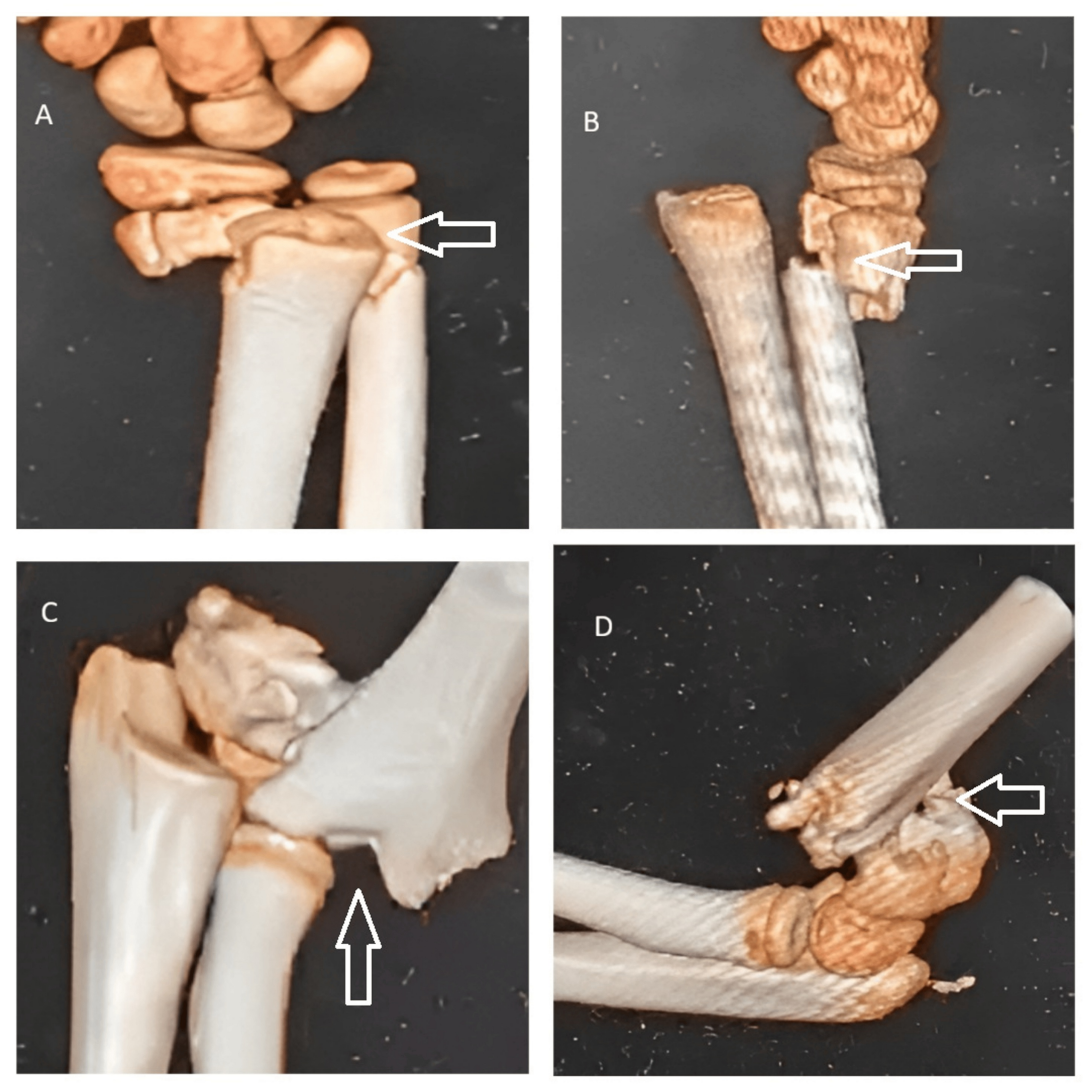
Preoperative CT images of the left elbow and wrist demonstrating fracture configuration Preoperative CT scans of the left upper limb showing metaphyseal fractures of the distal radius and ulna with minimal comminution (A,B) and a completely displaced, comminuted supracondylar humerus fracture (C,D). The images confirm extra-articular involvement and aid in surgical planning for fixation CT: computed tomography
